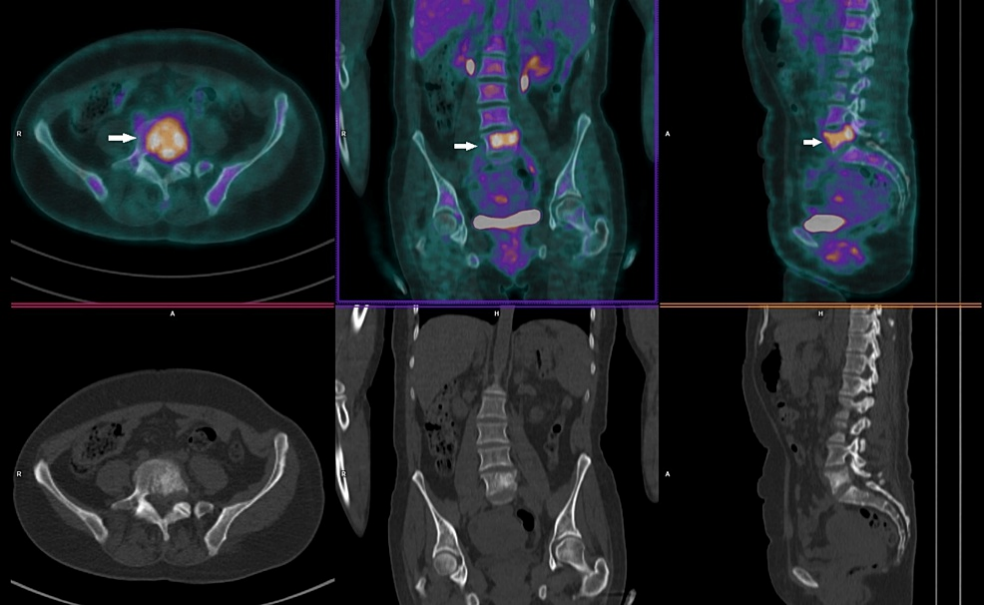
Staging 18F-FDG PET/CT scan. 18F-FDG PET/CT scan showing a hypermetabolic malignant lesion involving the body and left pedicle of the L5 vertebrae, with endocanal (left anterolateral) and foraminal (left conjugation hole L5-S1) insinuation related to the lesion described. There can also be seen a pathological fracture of this vertebral element. The remaining study did not show any other foci of anomalous radiopharmaceutical uptake that could suggest other hypermetabolic lesions.
